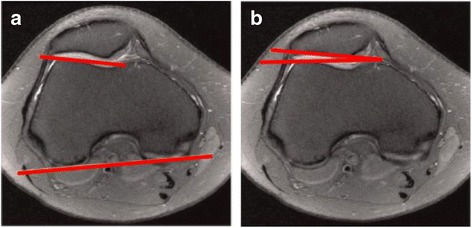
Method for measuring patellar tilt. Fulkersons angle (a) is the angle of the lateral facet of the patella, relative to the line connecting the posterior condyles on axial computed tomography/magnetic resonance imaging. Angle of Laurin (b) – the lateral patellofemoral angle assessed with the knee in 20° of flexion. This angle is formed by the lateral patellar facet and a line drawn across the most prominent aspect of the anterior portion of femoral trochlea
